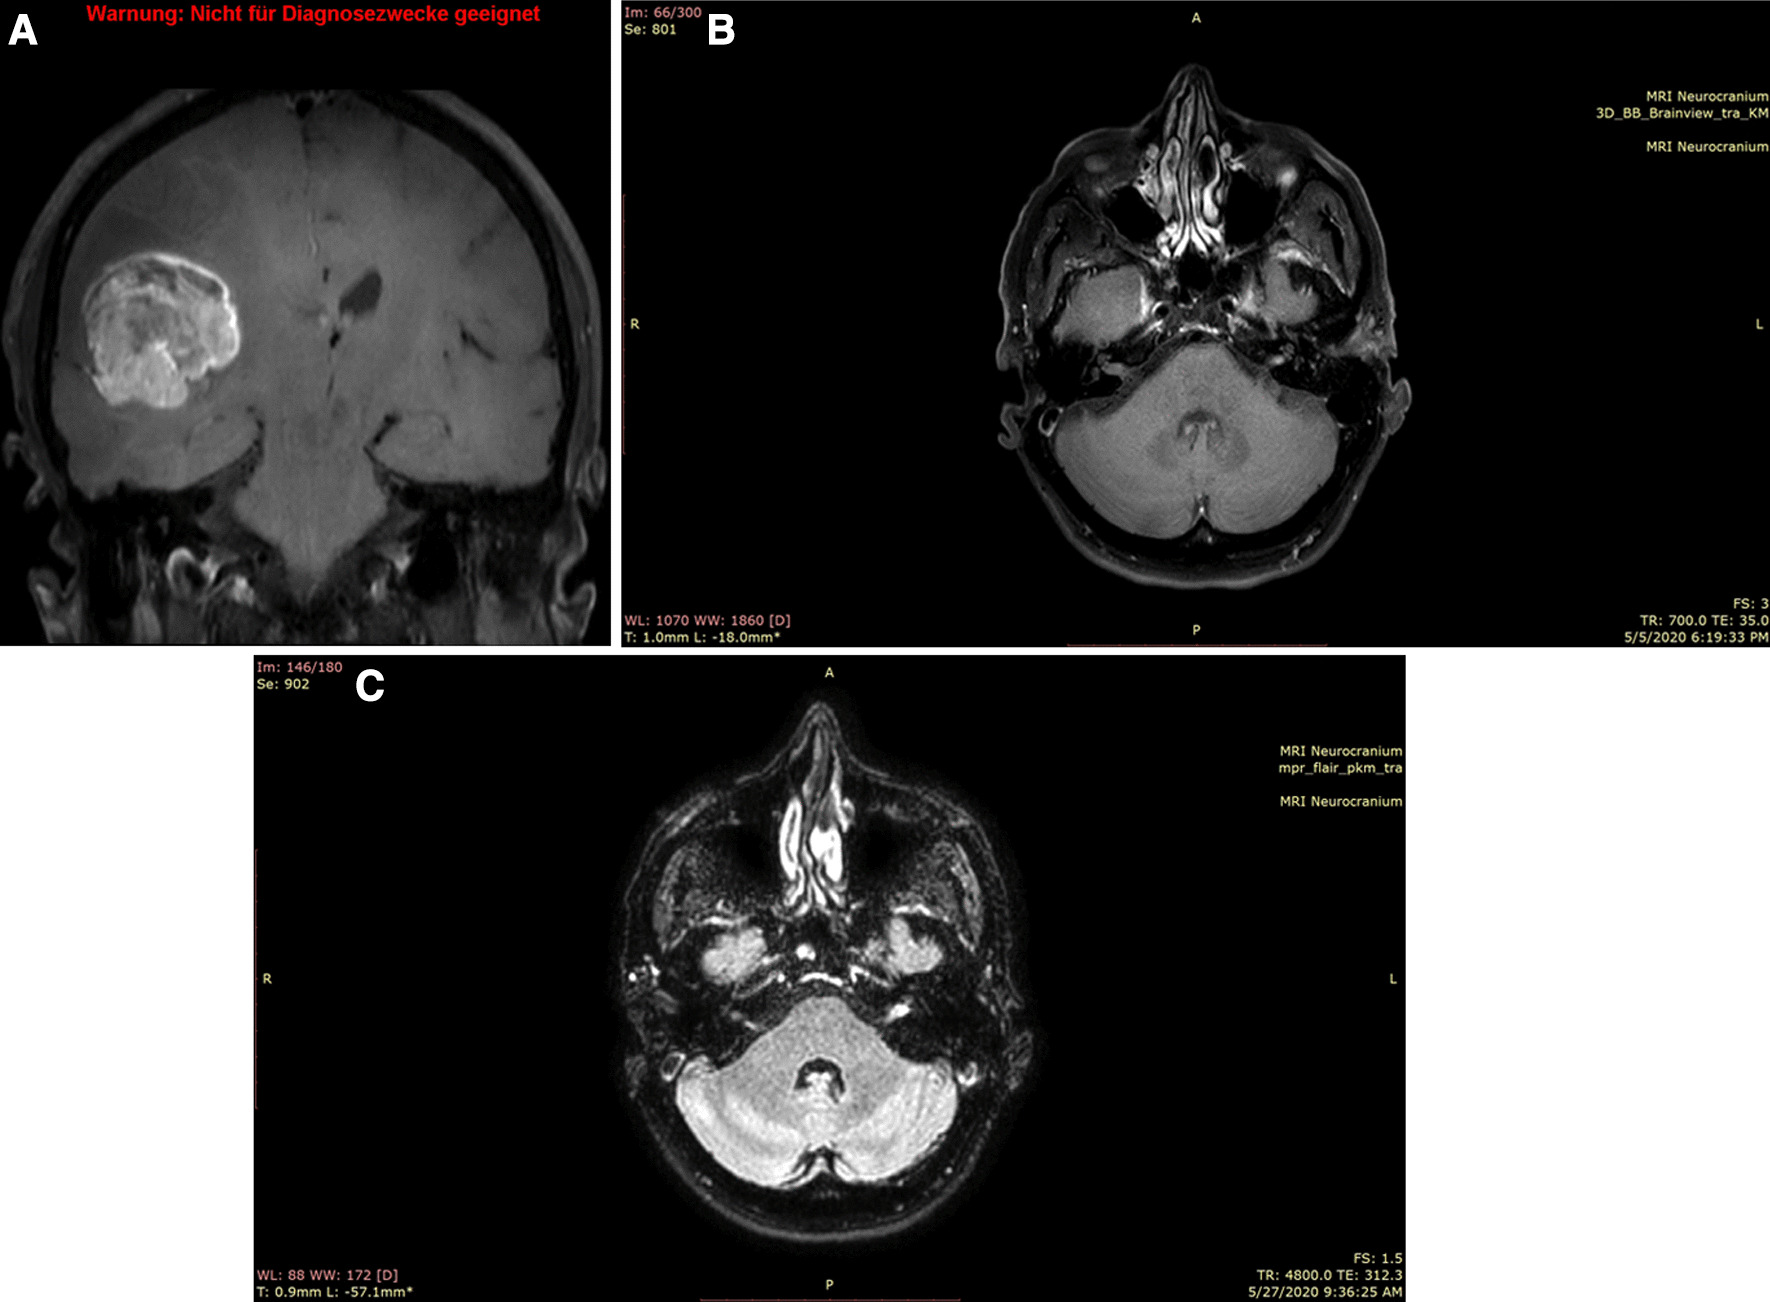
Brain magnetic resonance imaging at diagnosis and after resection of the tumor in the right temporoparietal lobe. a T1-weighted black-blood cMRI with contrast in transverse plane showing a tumor in the temporoparietal lobe at diagnosis (baseline). b T1-weighted cMRI after contrast showing a singular hyperintensity in the left internal acoustic channel at diagnosis (baseline). c Fluid-attenuated inversion recovery (FLAIR) image after contrast showing the lesion in the left internal acoustic channel after surgical resection of the right temporoparietal tumor
